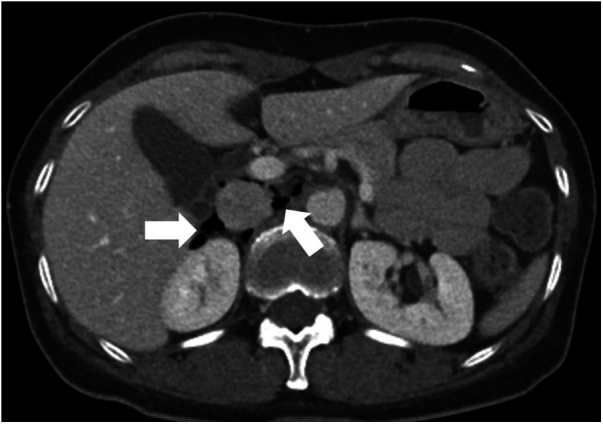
Abdominal CT findings suggestive of a spontaneously sealed duodenal microperforation. The image reveals minimal periduodenal pneumoperitoneum (asterisk).
